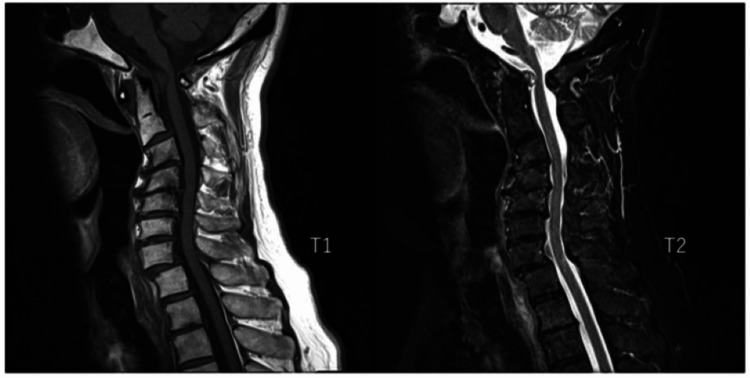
Preoperative MRI A retro-odontoid mass lesion with an iso- and a high-intensity in T1- and T2-weighted images, respectively.
